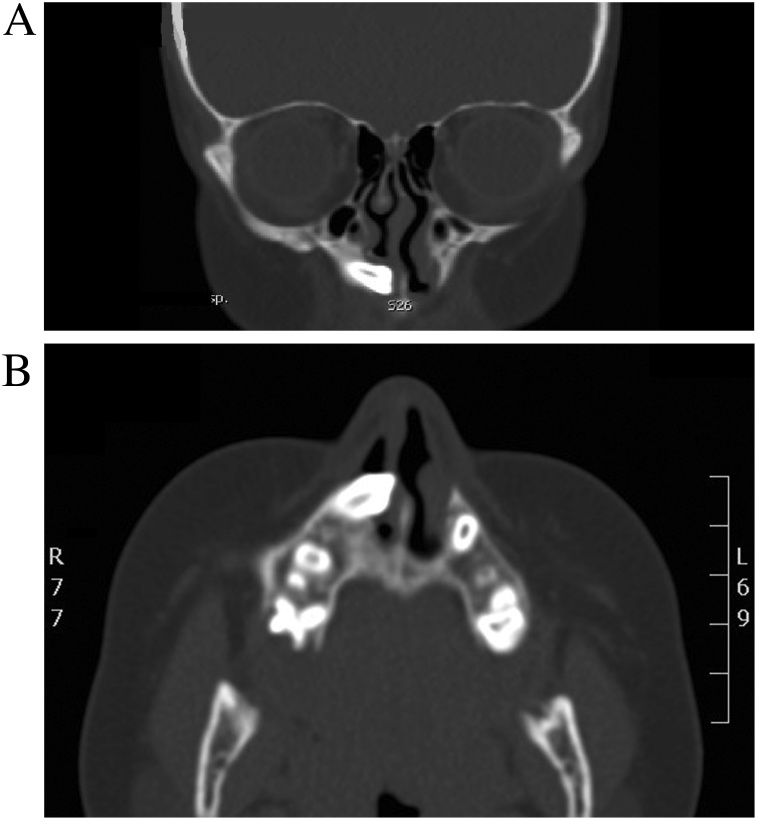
A,B: Computed tomography of the paranasal sinuses (coronal and axial view) revealing displaced right upper maxillary tooth with the crown oriented inferiorly and medially toward and within the lower right anterior nasal cavity.
